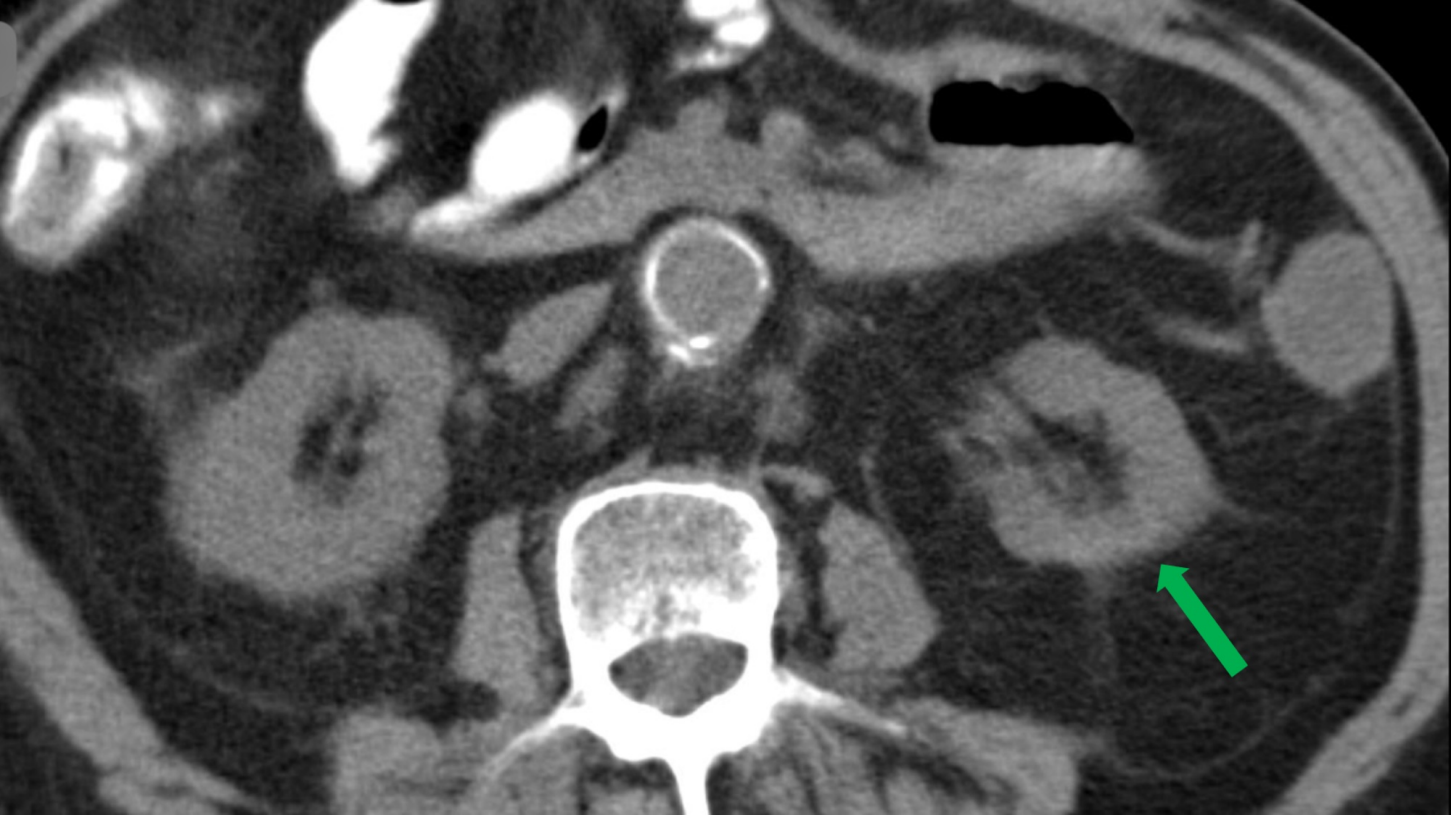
Four-year post-embolization follow-up axial CT showing no residual tumor in the left kidney (green arrow). Abbreviation: CT, computed tomography.
